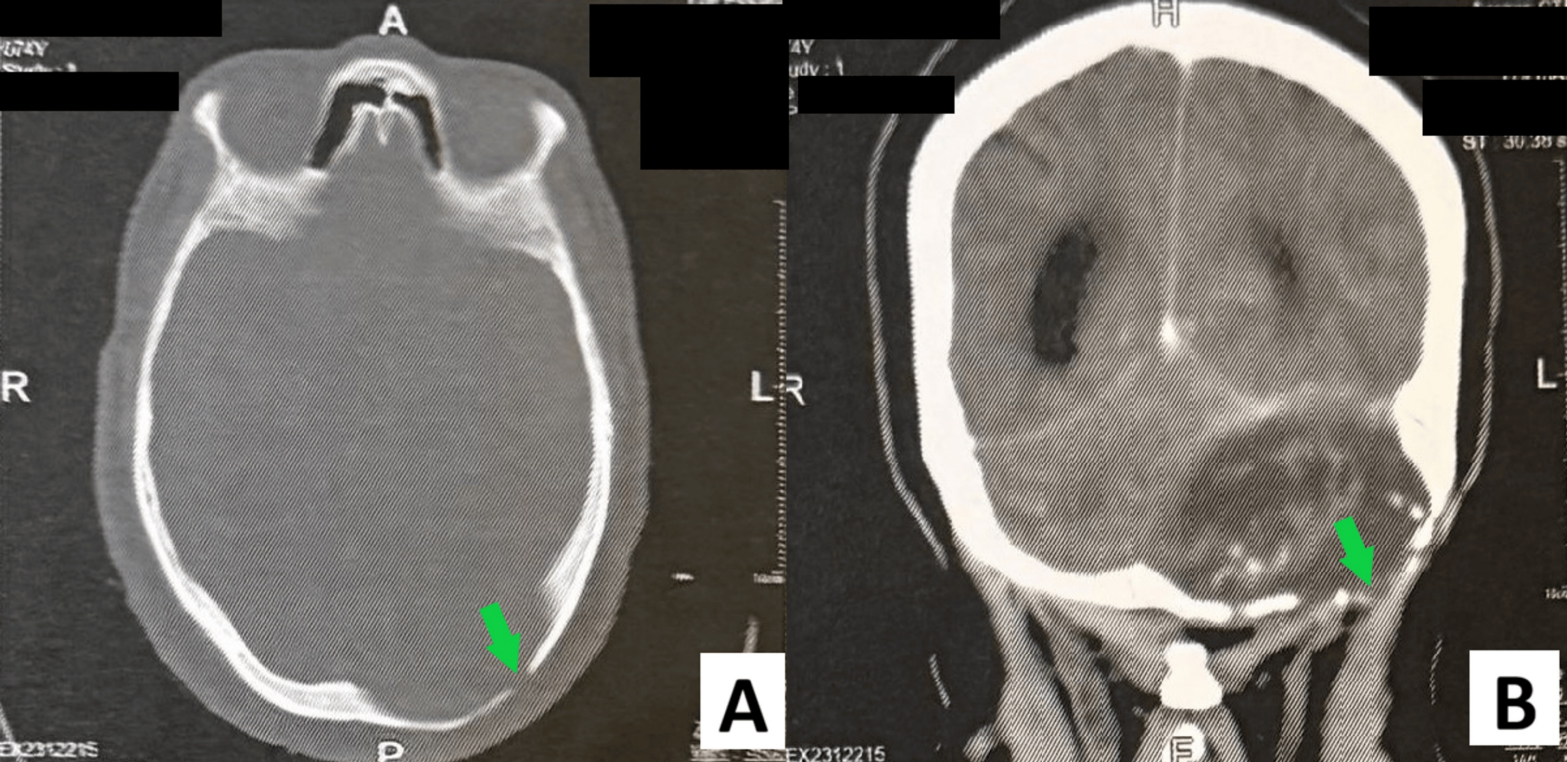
Preoperative CT scan findings. A: Axial CT scan bone reconstruction demonstrating extensive osteolysis and thinning of the left occipital bone (green arrow), consistent with a long-standing, slowly progressive lesion. B: Coronal non-contrast CT scan showing a large hypodense extra-axial lesion occupying the left posterior cranial fossa, exerting mass effect on the adjacent cerebellar hemisphere.
